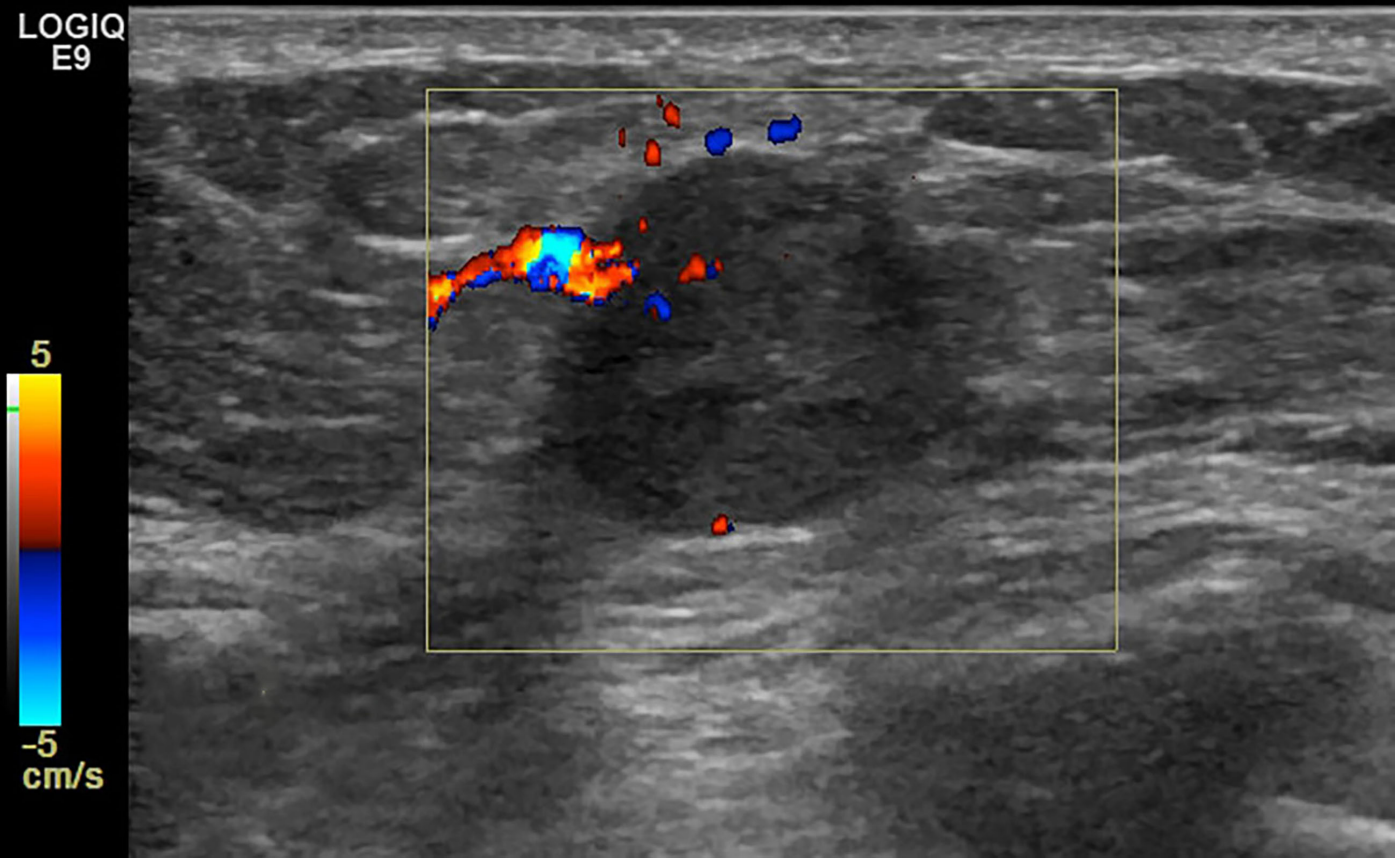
Case 3: A 49-year-old woman with cPMBC presented with a hypoechoic mass (1.7 × 1.4 × 1.3 cm) in the right breast. The lesion presented with linear blood flow signal by color Doppler mode.
